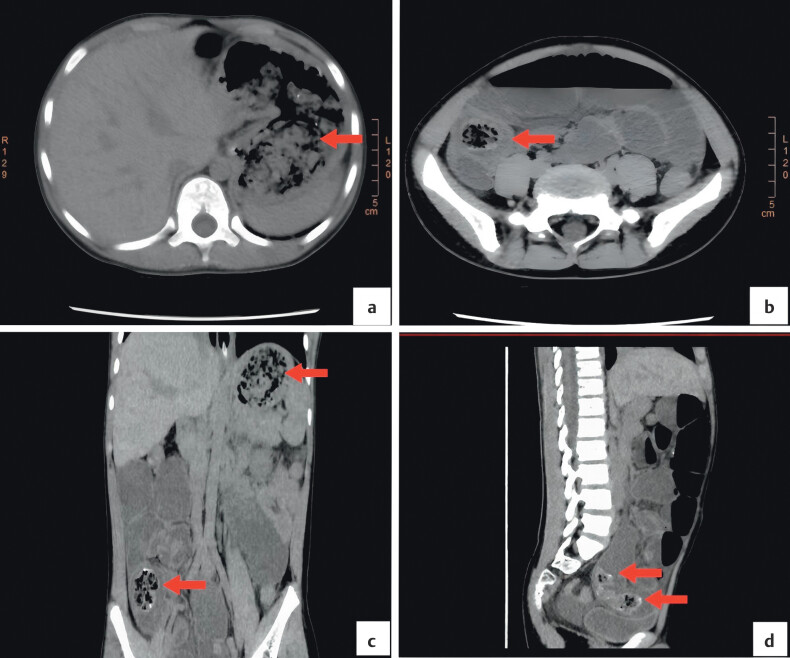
Abdominal CT is showing a huge gastric bezoar (5.7×4.5×6.0 cm)
